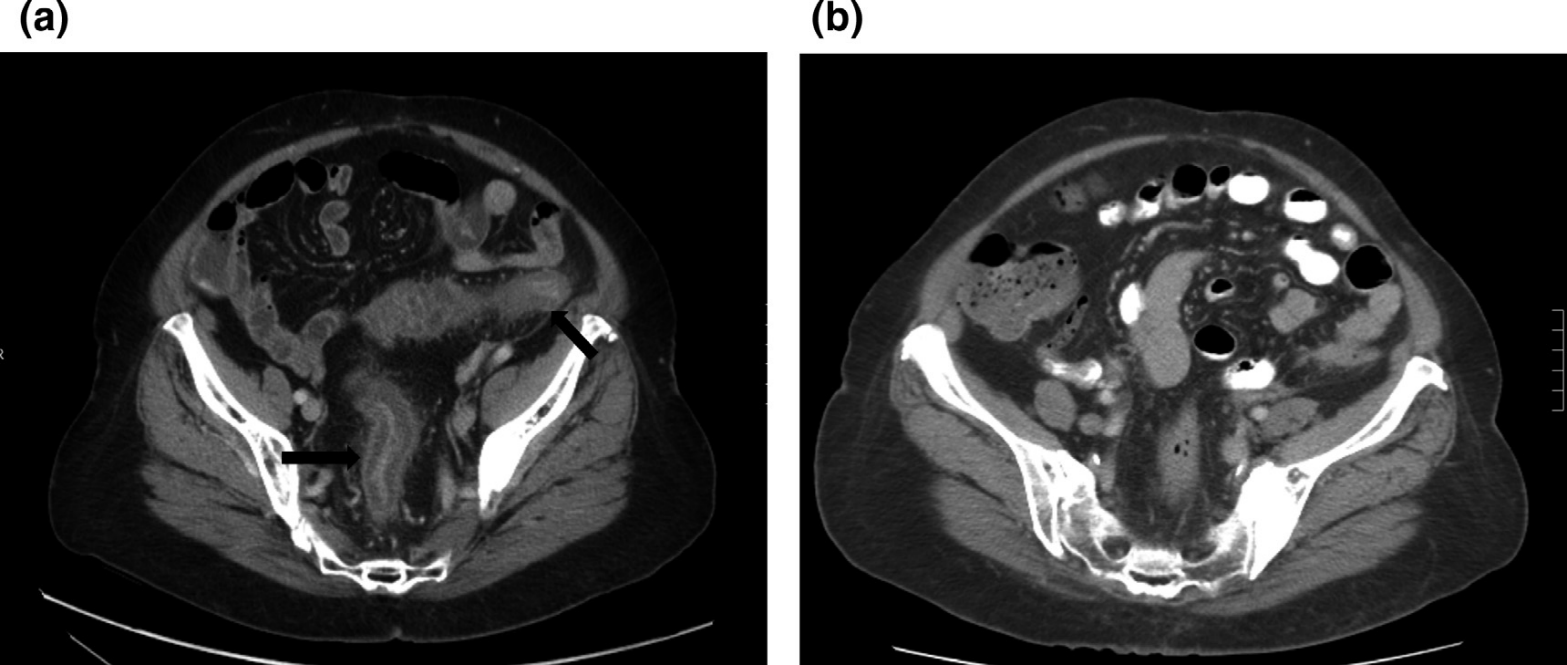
Figure A is the computed tomography axial view done at 5 days after completion of radiation (during admission) showing colitis represented as thickening of mucosa most apparent in the descending colon and rectosigmoid junction (arrows). Figure B shows the repeat computed tomography at 3 weeks from radiation completion with a resolution of the mucosal thickening.
